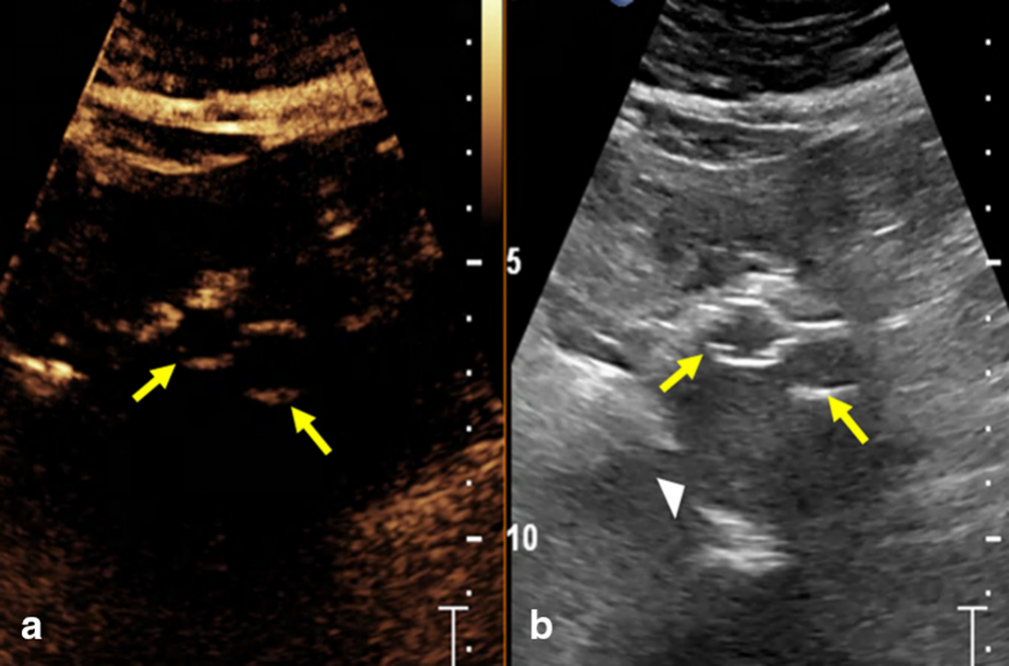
Transverse pre-contrast ultrasound image (a) and grayscale image (b) at the level of the infrarenal abdominal aortic aneurysm status post-endovascular aneurysm repair and coil embolization of the right L2 and L3 lumbar arteries. The echogenic iliac artery stent grafts (arrows) are readily visible on both ultrasound images. The aneurysm sac is anechoic and measures 5.1 cm (arrowhead).
